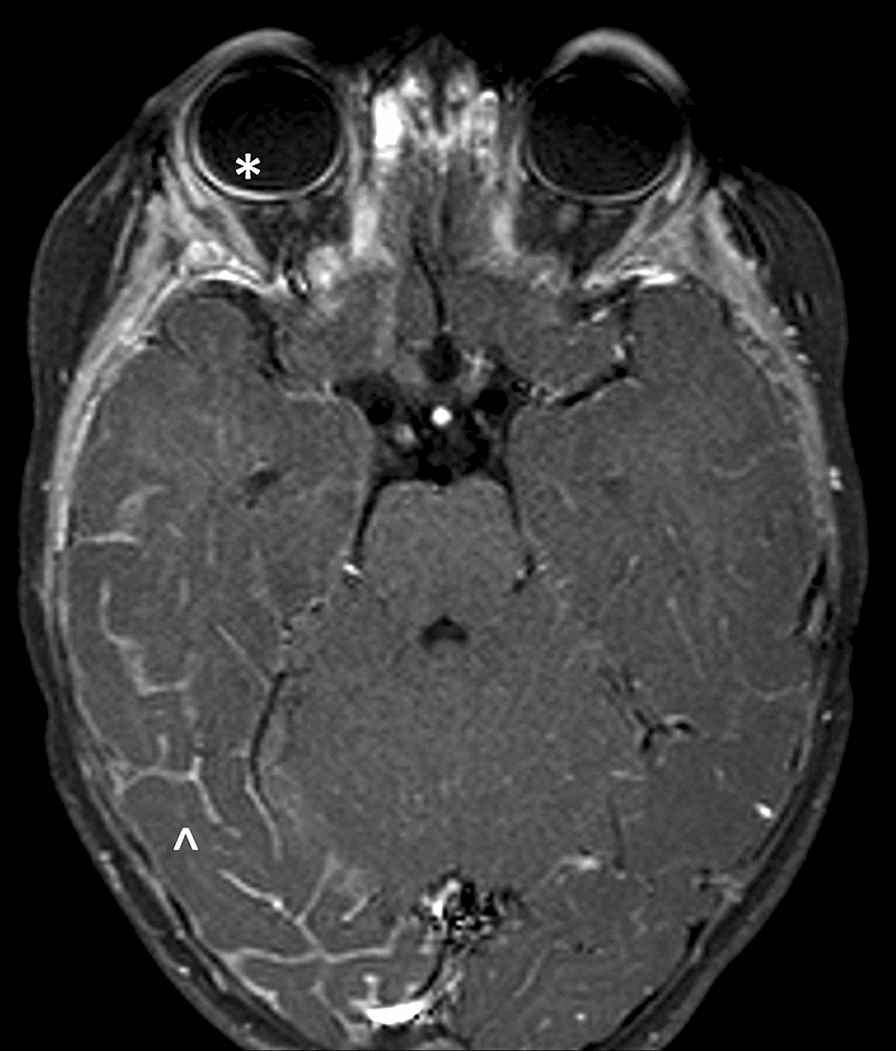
Gadolinium-enhanced axial T1-weighted brain MRI image in a child with Sturge–Weber syndrome. A prominent leptomeningeal enhancement of the right temporo-occipital lobes is evident (^); a homolateral choroidal vascular malformation is also visible into the ocular globe (*), compared to contralateral eye
